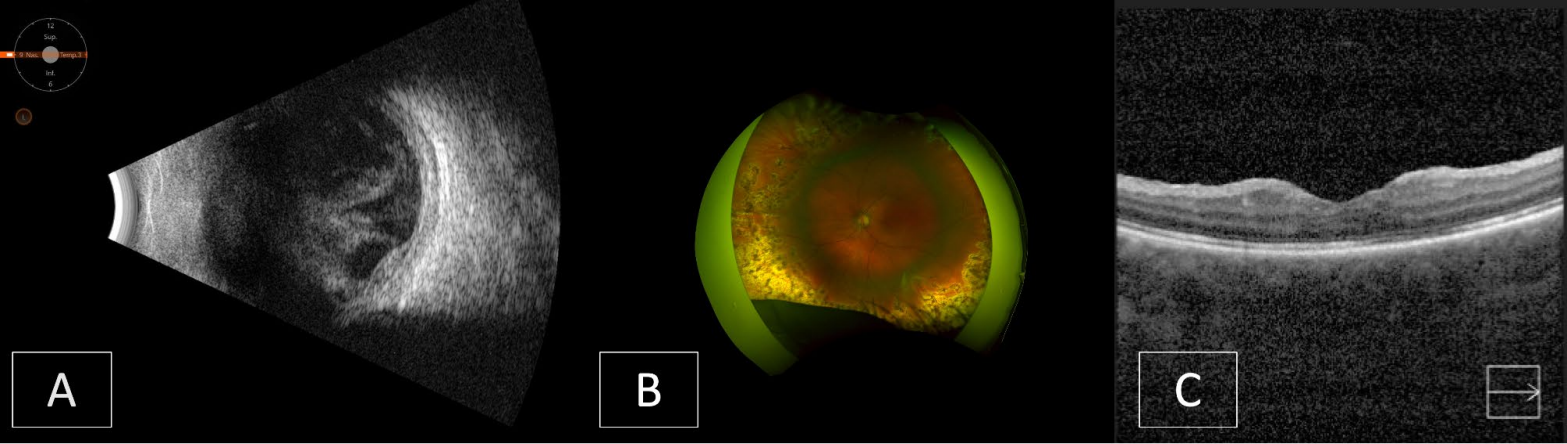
Case 1 images. A: B-scan ultrasound following the open globe repair. B: Optos image following the third pars plana vitrectomy. C: Optical coherence tomography one year post-trauma
